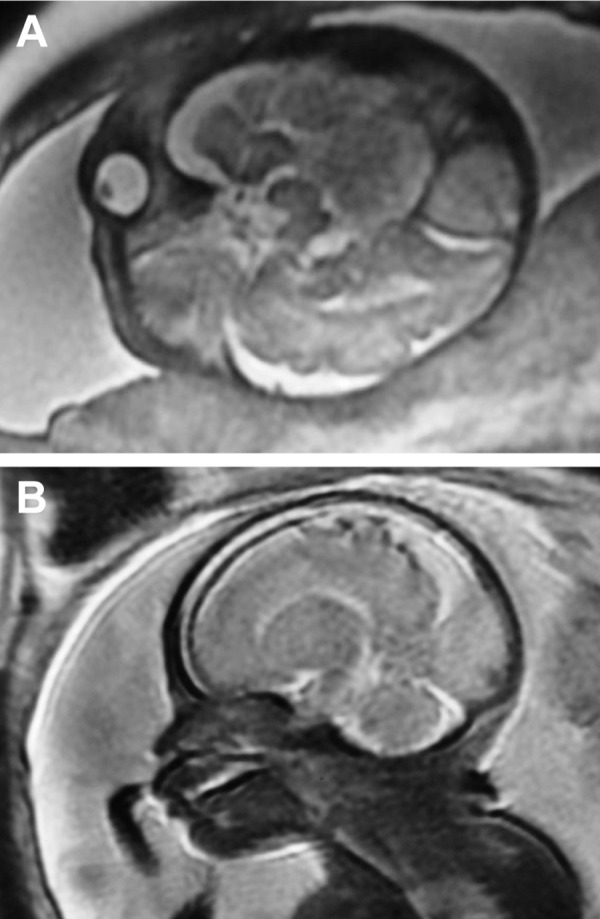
MRI images of the fetal head at 32 weeks’s gestation shows a triangle dural sinus thrombosis measuring 2.5×2.4cm at torcular. A. T2 weighted image at axial plane with high signal intensity, B. T2 weighted image at sagittal plane shows dilation of the superior longitudinal sinus.
